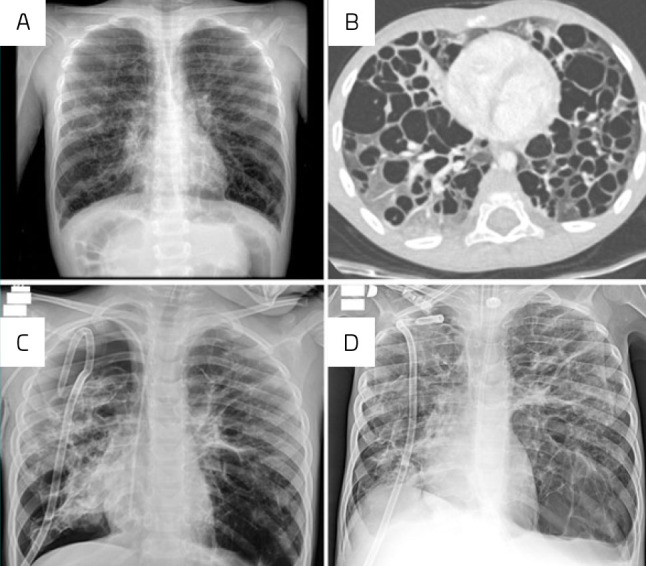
(A) An initial chest X-ray led to the diagnosis of pneumonia. (B) Chest CT showed multiple and disseminated pulmonary cysts throughout the lung parenchyma. (C) Chest X-ray after draining the right hemithorax. (D) A new chest X-ray depicting enlargement of the bubble on the left base, with mediastinal deviation to the right side.
